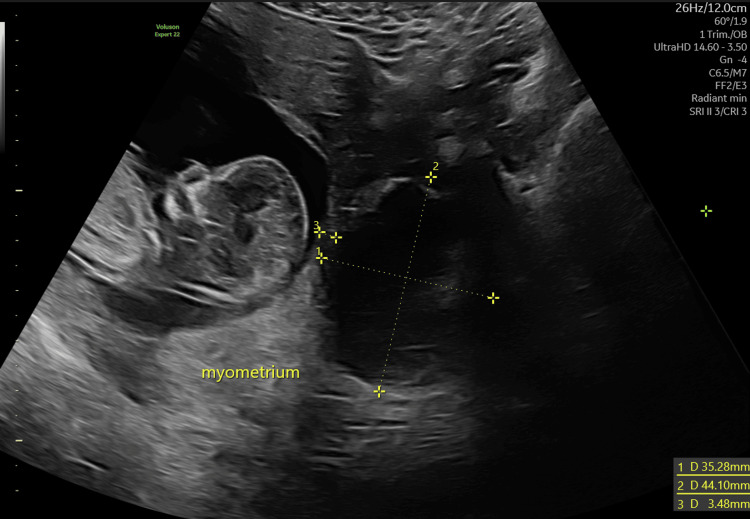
Transabdominal ultrasound at 13 weeks + two days (first trimester), transverse uterine view showing a viable intrauterine pregnancy and a predominantly anechoic mass with two orthogonal measurements and its distance from the amniotic cavity.
